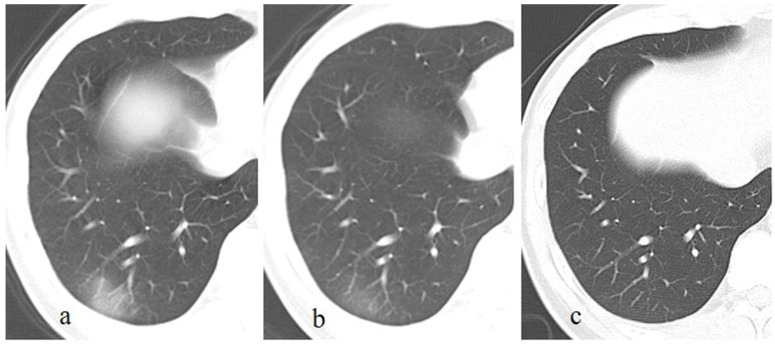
A patient with a history of travelling to Wuhan recently, presents with fever and cough for 13 days. The initial chest CT at the first day after admission shows a patchy GGO in the right lower lobe (a). Subsequently (5 days later), this lesion is directly absorbed with decrease of extent and density (b). On the latest CT scan (18 days later), the lesion is completely absorbed (c).
